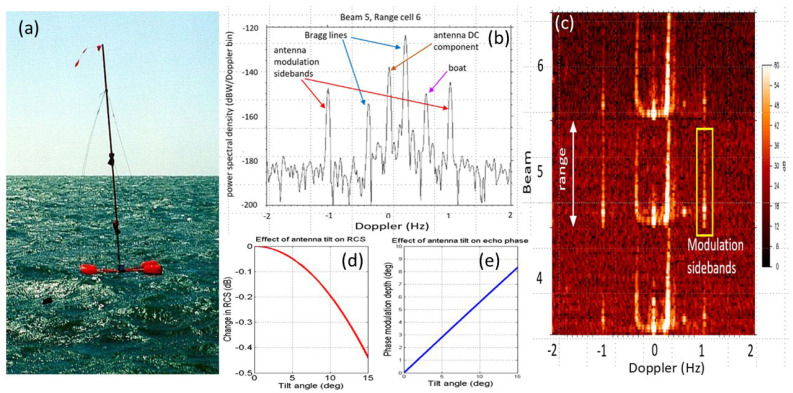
(a) A switched impedance buoy, (b) the Doppler spectrum it presents in a single range bin, (c) the signature distributed across a range–Doppler map, and (d) plots showing the sensitivity of RCS and phase to tilt.
